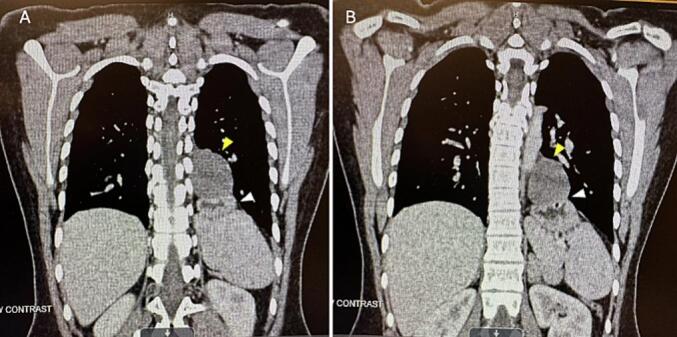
Chest computed tomography with contrast preoperative. Moderate to large left diaphragmatic/hiatal hernia (white arrow). Adjacent 4.3 cm lower mediastinal cyst (yellow arrow) most likely representing benign pathology such as bronchogenic or pericardial cyst. (For interpretation of the references to colour in this figure legend, the reader is referred to the web version of this article.)
